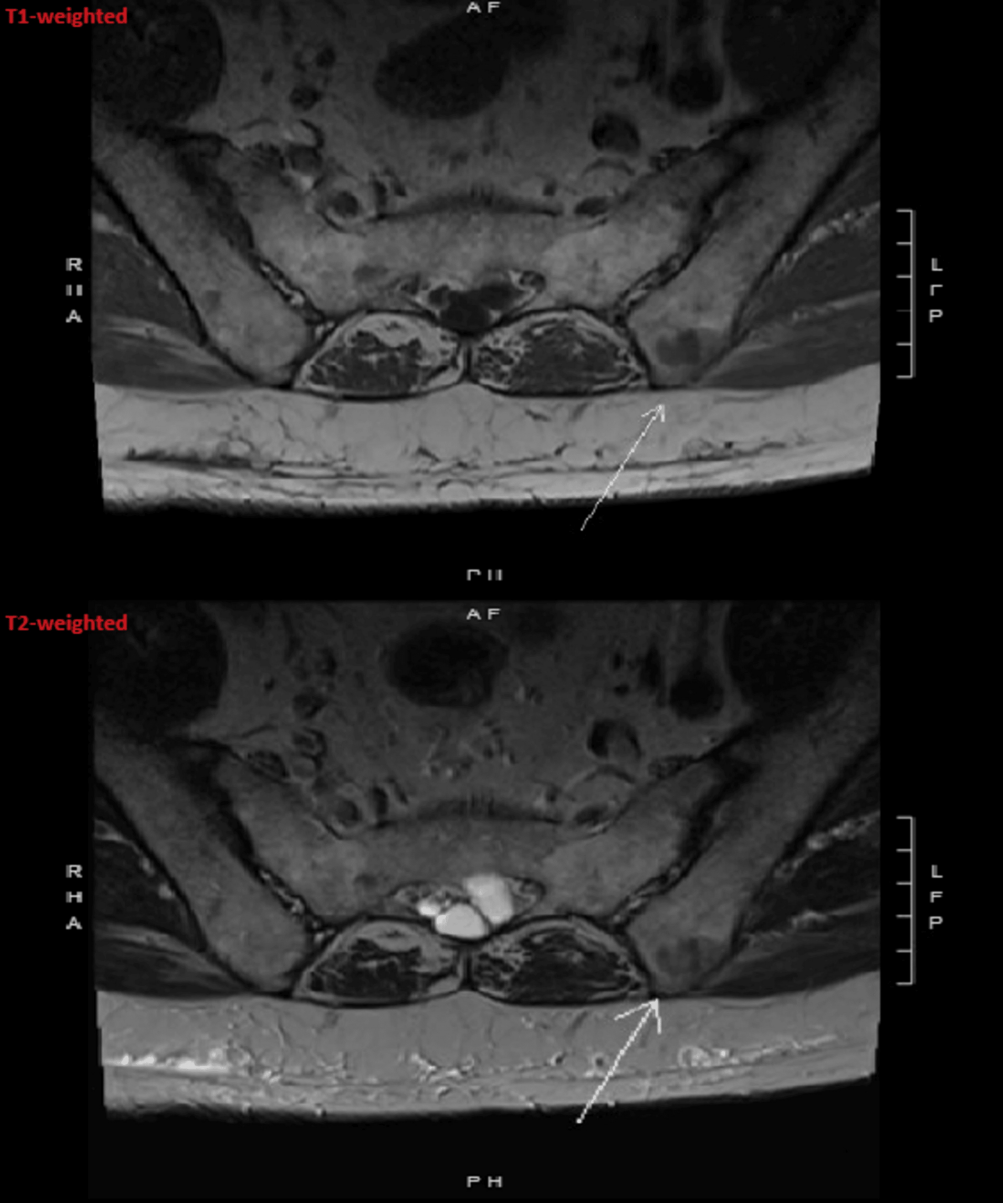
Magnetic resonance imaging of the lumbar spine without contrast demonstrating a lesion with low signal intensity on both T1-weighted and T2-weighted sequences involving the iliac bone (marked by the white arrow).
